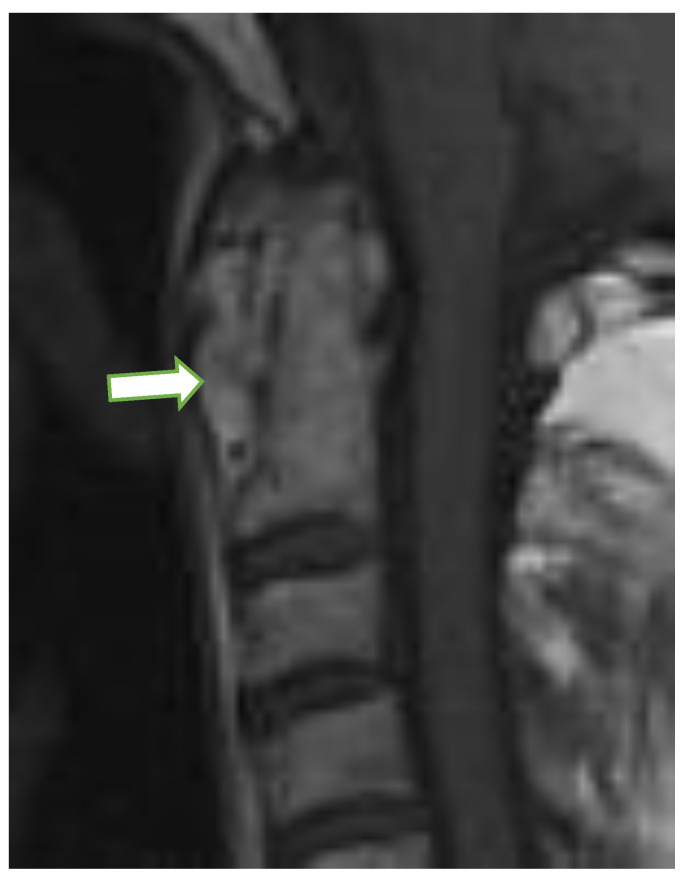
Sagittal postcontrast MRI, T1-w image in a 76-year-old female with rheumatoid arthritis with pannus formation in periodontal area (arrow). MRI: magnetic resonance imaging.
